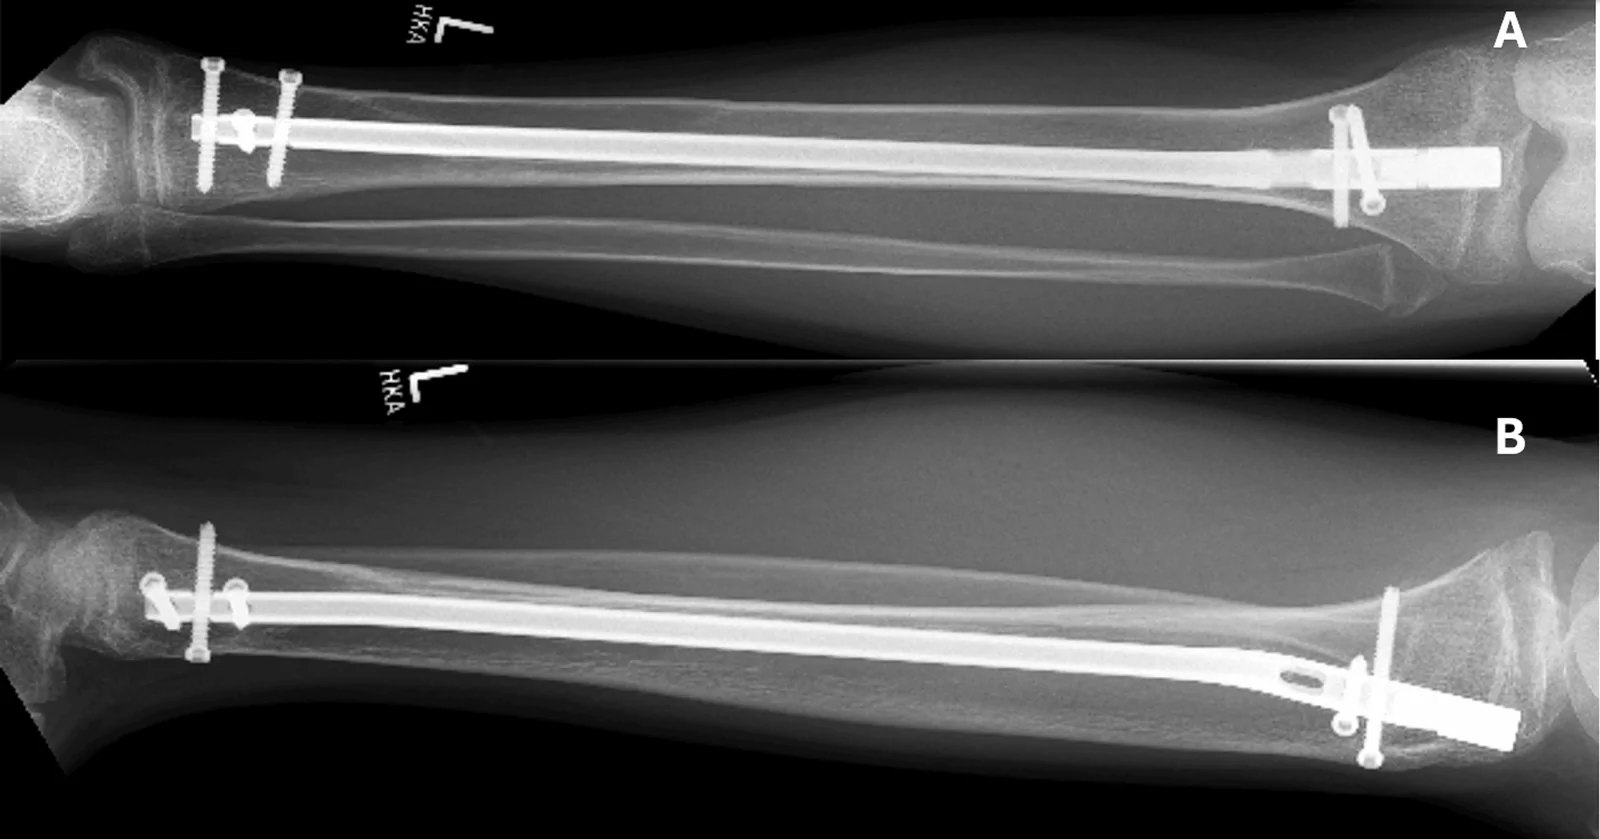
Postoperative radiographs of the left lower leg showing intramedullary interlocking nail fixation with satisfactory fracture alignment and hardware position. (A) Anteroposterior view; (B) Lateral view
